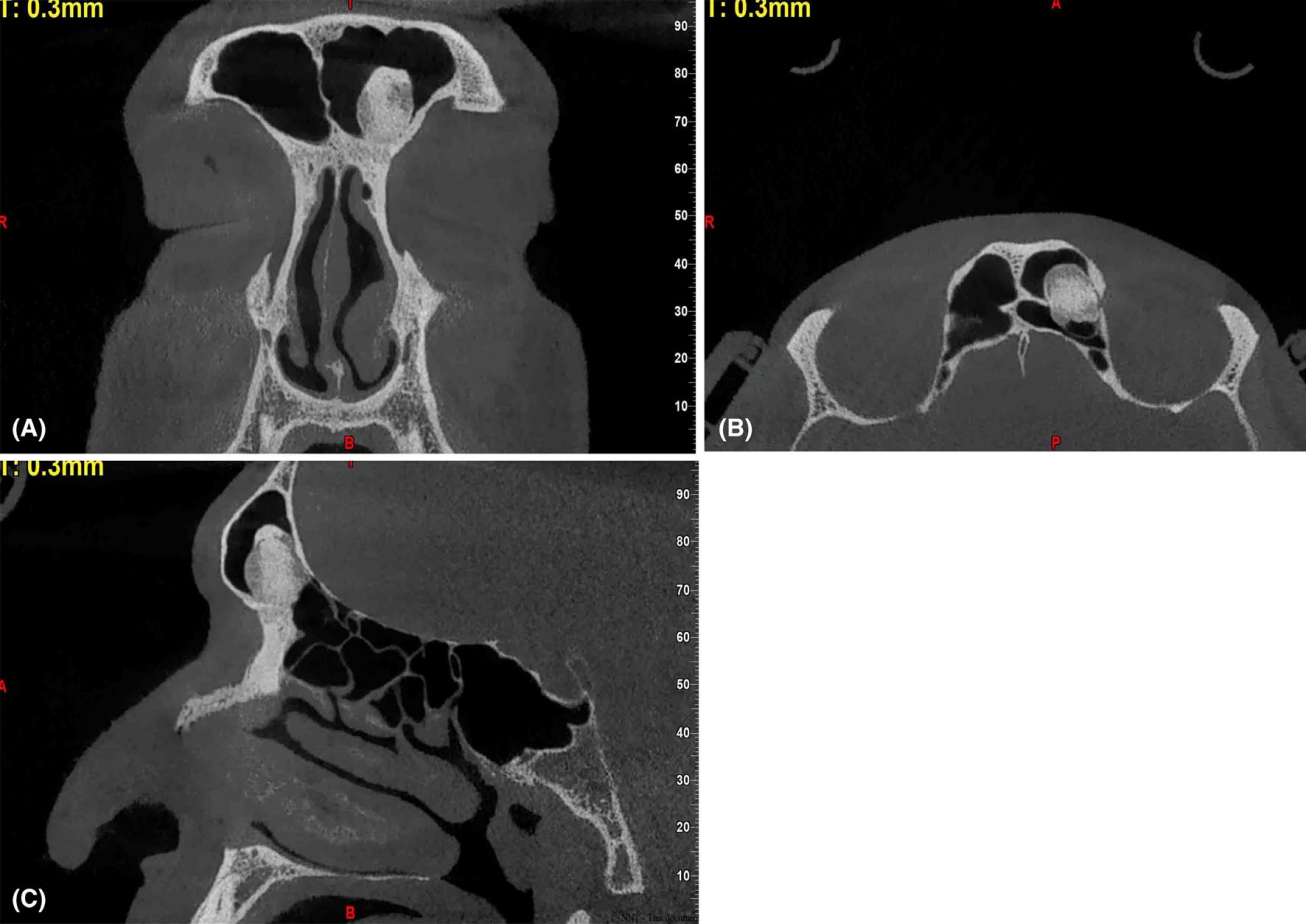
(A) Coronal and (B) axial and (C) sagittal cone‐beam computed tomography scan revealing a dense radiopaque mass in left frontal sinus.
